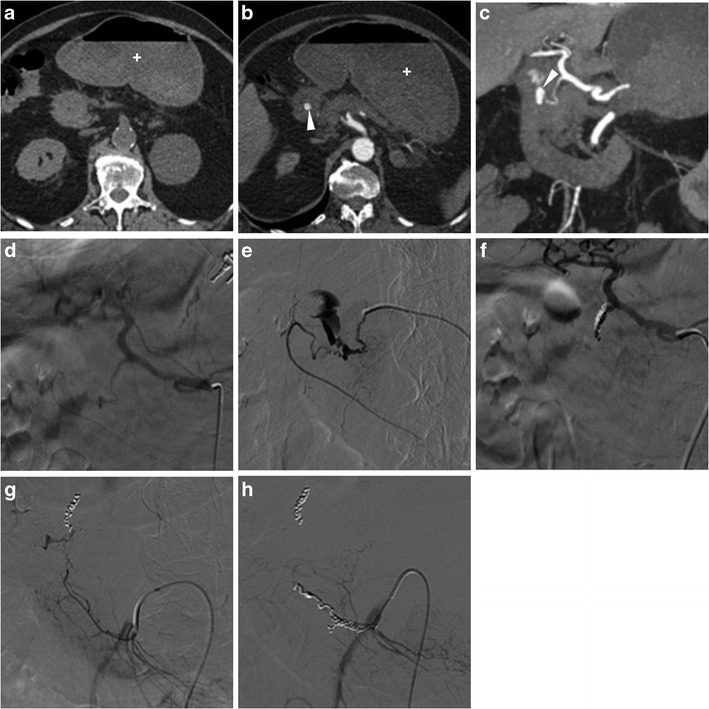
A 63-year-old, haemodynamically unstable (blood pressure 70/40 mmHg) female with massive haemoptysis (haemoglobin 5 g/dl) underwent CT-angiography following inconclusive endoscopy. Precontrast images (a) showed bloody fluid (+) in the stomach. Arterial-phase images (a) and Maximum-intensity projection (MIP, b) reconstructions revealed a focal contrast “blush” extravasation within the duodenal lumen (arrowheads) originating from the gastroduodenal artery (GDA). Angiography (d) and selective angiography (e) confirmed active bleeding from the GDA. Despite coil embolisation of the GDA (f) having obtained apparent exclusion of haemorrhage, angiography of the superior mesenteric artery (g) showed refilled bleeding through a branch of the arc of Buhler. Finally, after repeated embolisation on that second vessel, angiography (h) showed complete resolution of haemorrhage
